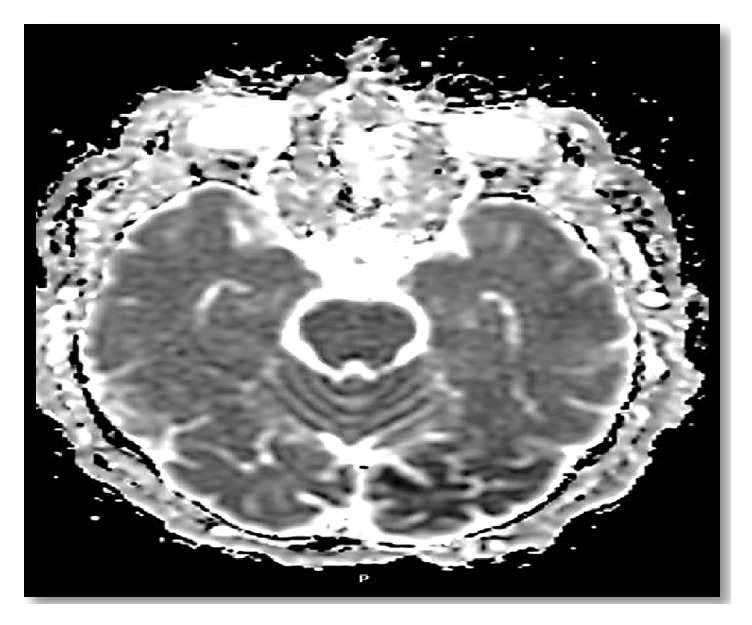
Periictal brain MRI ADC mapping showing bilateral occipital hypointense signal alteration (left>right). Abbreviations: ADC, apparent diffusion coefficient; MRI, magnetic resonance imaging.
